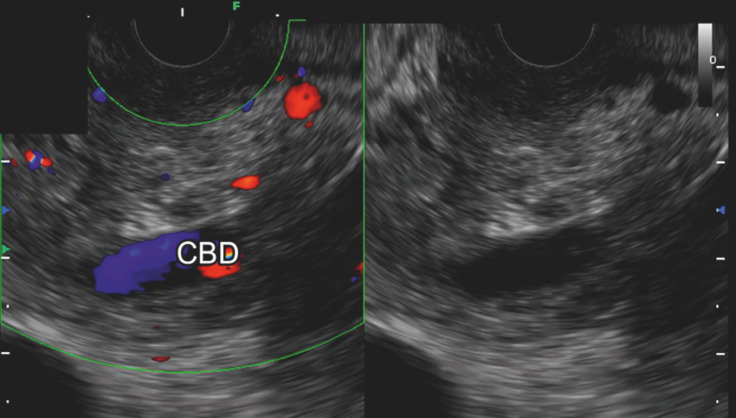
A turbulent flow signal was observed in the common bile duct after endoscopic ultrasound-guided fine needle aspiration.
